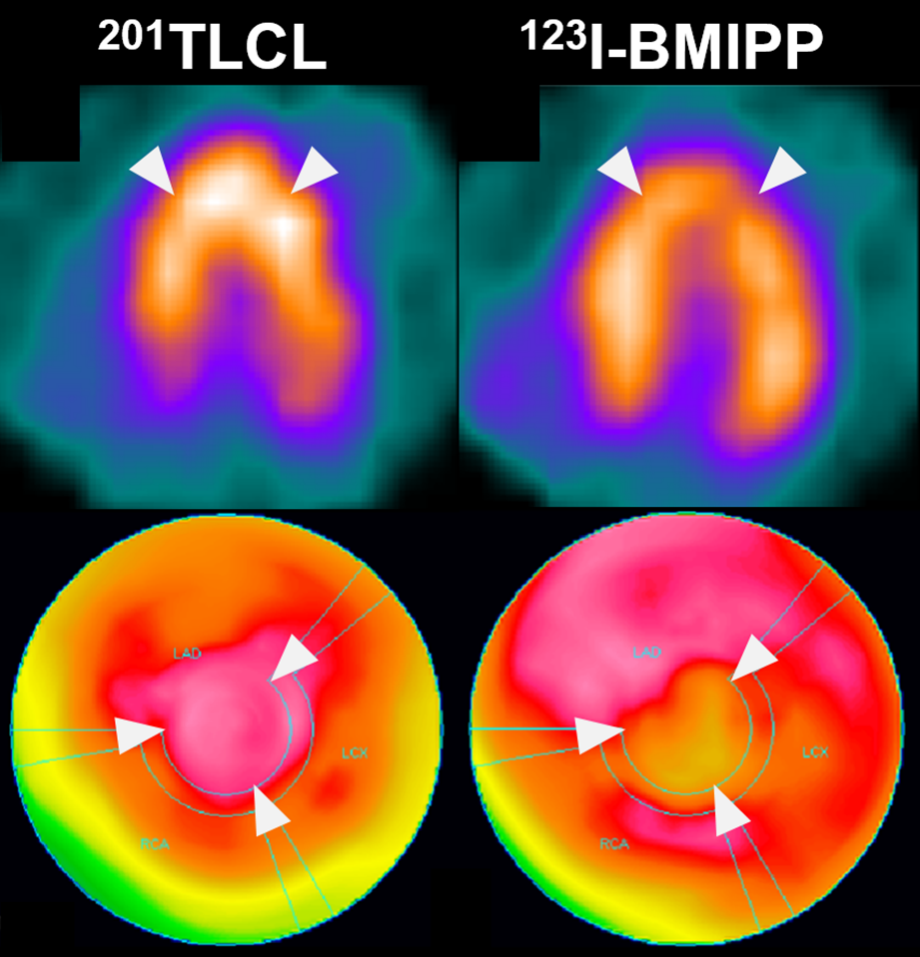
Dual isotope scintigraphy images at four days after admission showing increased ^201^TLCL uptake and decreased ^123^I-BMIPP uptake at LV apex (white arrow). This mismatch of nuclear uptake findings suggested tako-tsubo-like LV dysfunction. ^201^TLCL, thallium-201 chloride; ^123^I-BMIPP, iodine-123-beta-methyl-p-iodophenyl-pentadecanoic acid
